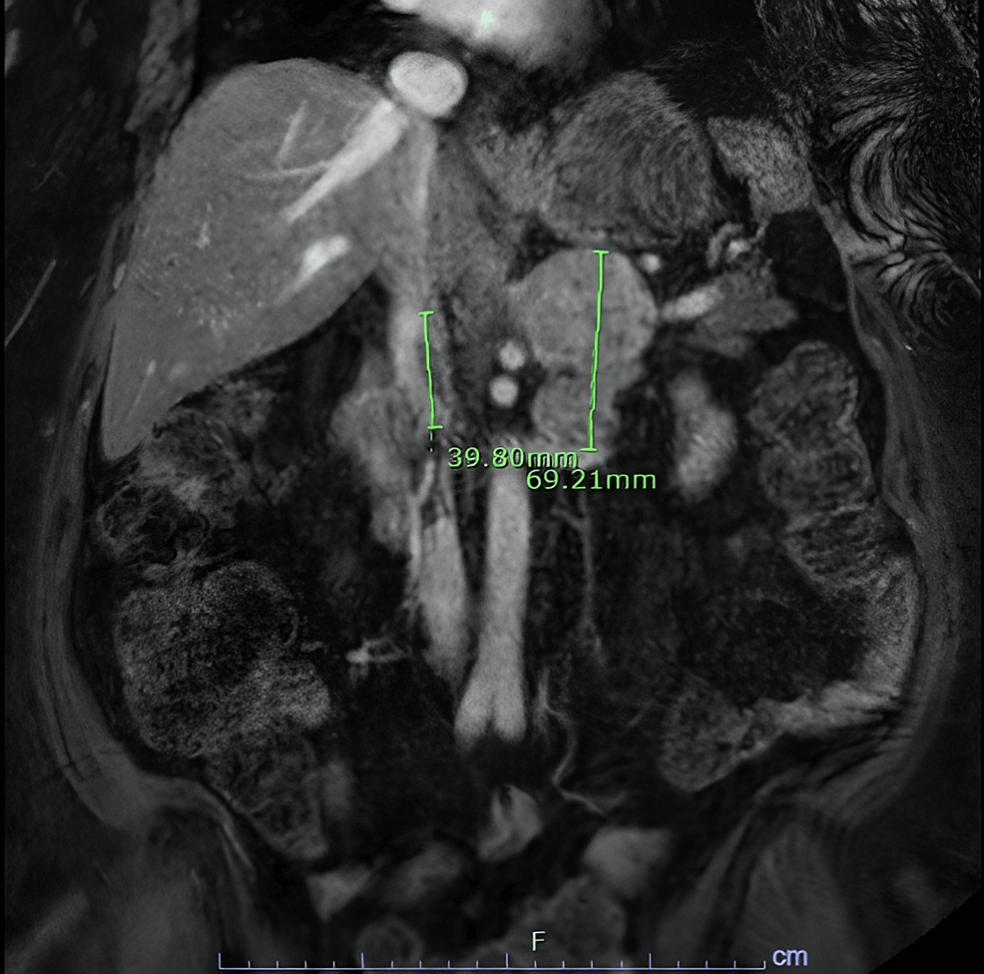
Adrenal MRI with contrast (coronal view) The left mark shows a nonocclusive thrombus in the IVC, and the right mark shows a 69.21 mm bilobated adrenal mass with tumor invasion to the left renal vein.
